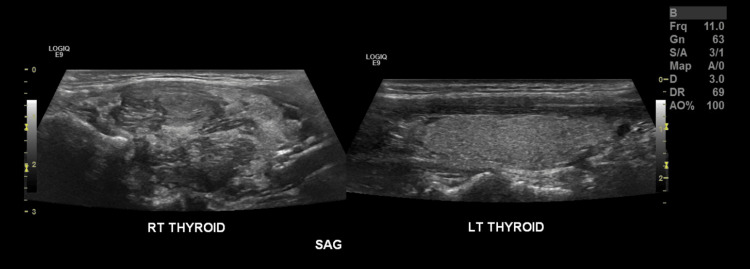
Sagittal ultrasound of the right vs left thyroid. RT THYROID: right thyroid; LT THYROID: left thyroid; SAG: sagittal
